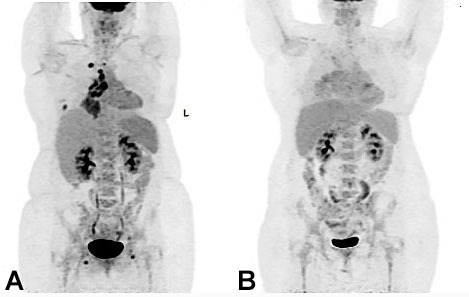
MIP PET images performed prior to the administration of Atezolizumab (A) and around 4 months later (B) demonstrate resolution of the FDG avid mediastinal and hilar lymph nodes, with almost complete resolution of the right apical and lower lobar nodules. L: Left.
